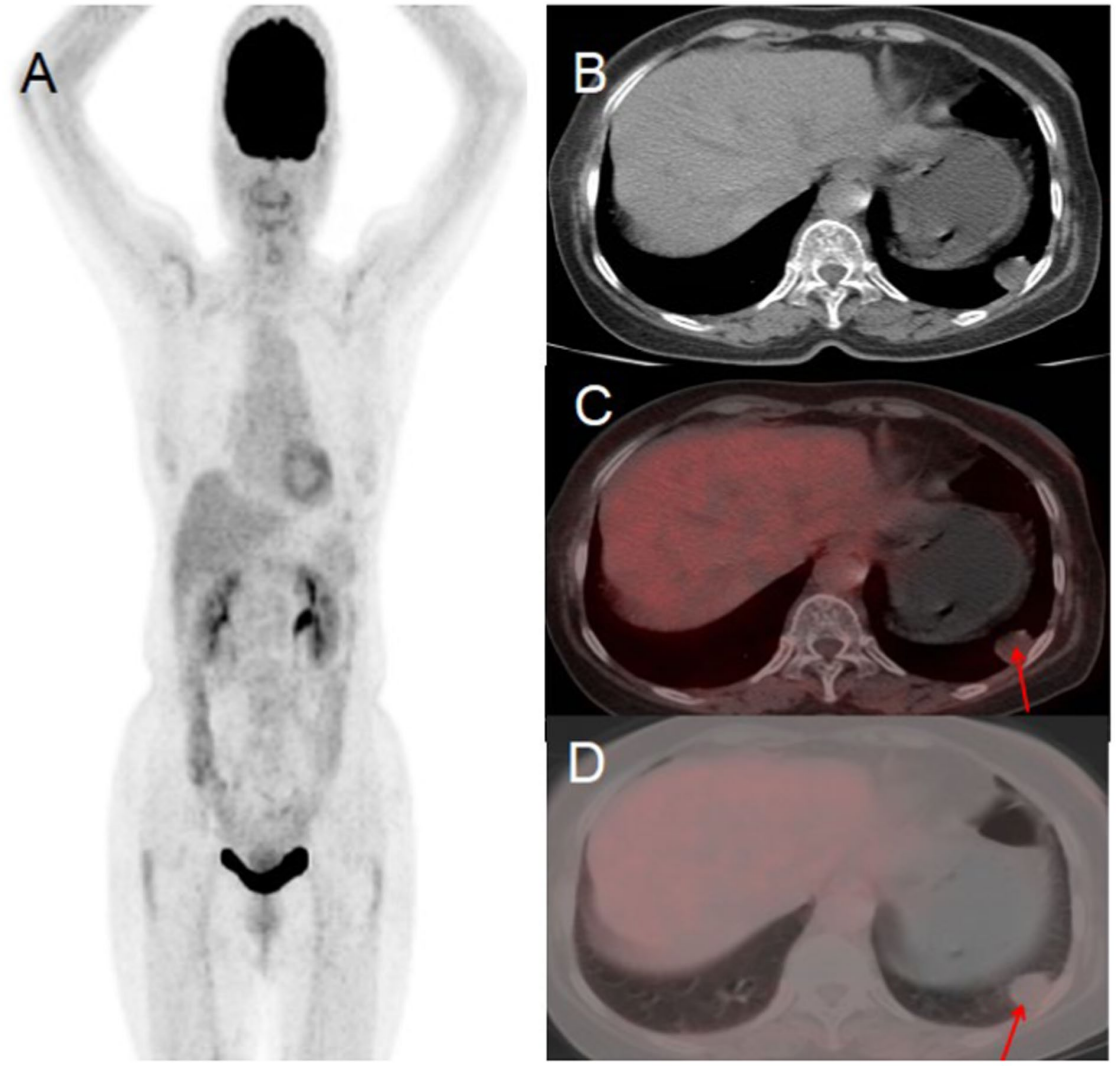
Female, 76 years old, benign primary pulmonary solitary fibrous tumors. (A) Whole body MIP. (B) Axial CT. (C) Axial fusion. (D) Axial fusion. PET/CT suggested a round lesion in the left lower lobe with mild FDG uptake, comparable to the background FDG uptake of the adjacent lung tissue (arrow, D).
